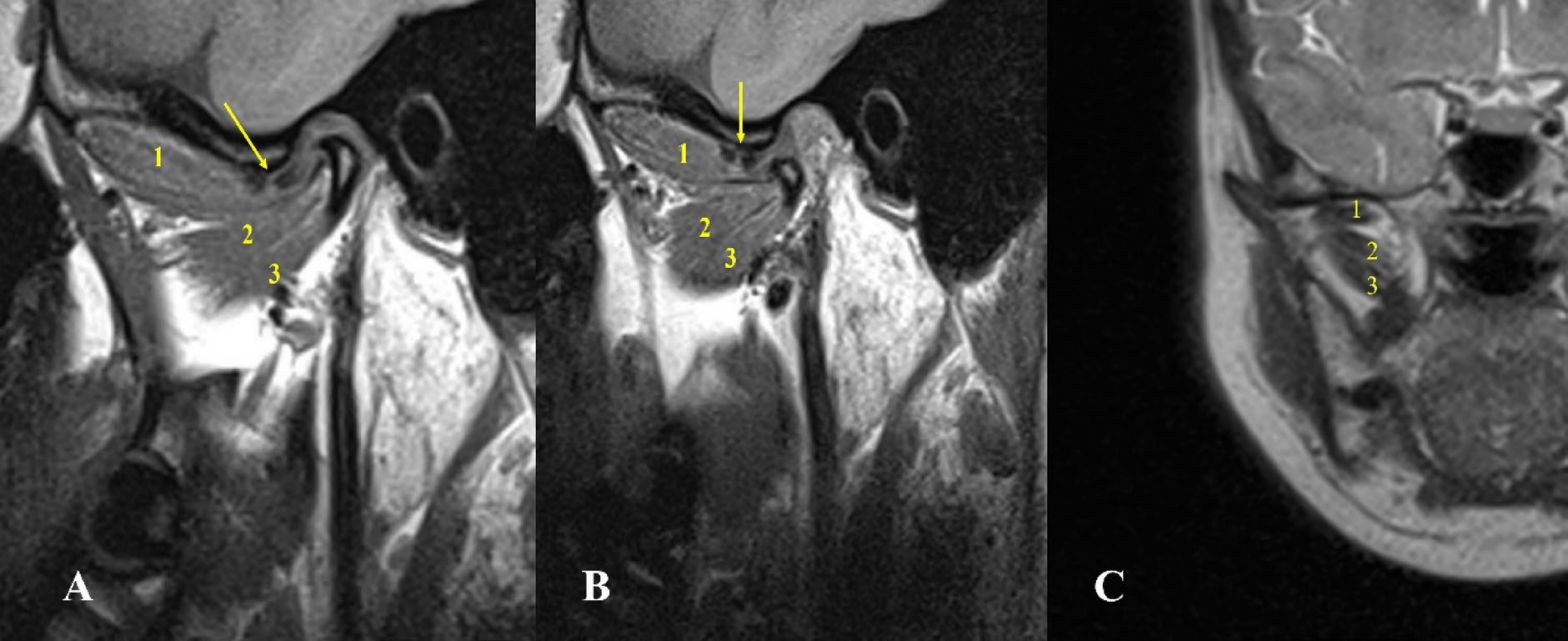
Oblique sagittal T1-weighted images represent Type III-A with three heads of lateral pterygoid muscle, the upper attached to the disc and the lower two attached to the condyle associated with disc displacement without reduction and condylar erosion, osteophyte and condylar resorption.
